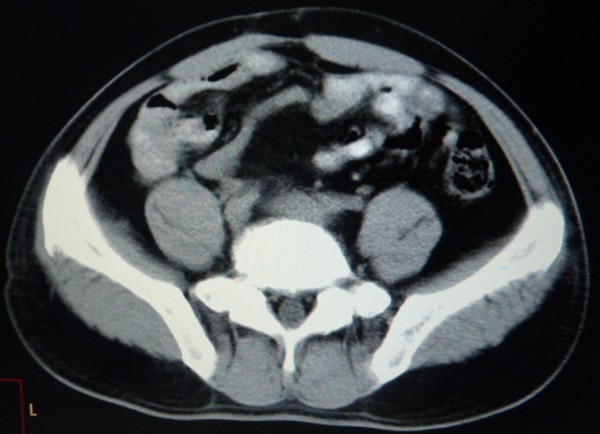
CT Imaging in follow-up within fifth year. CT, computed tomography.
